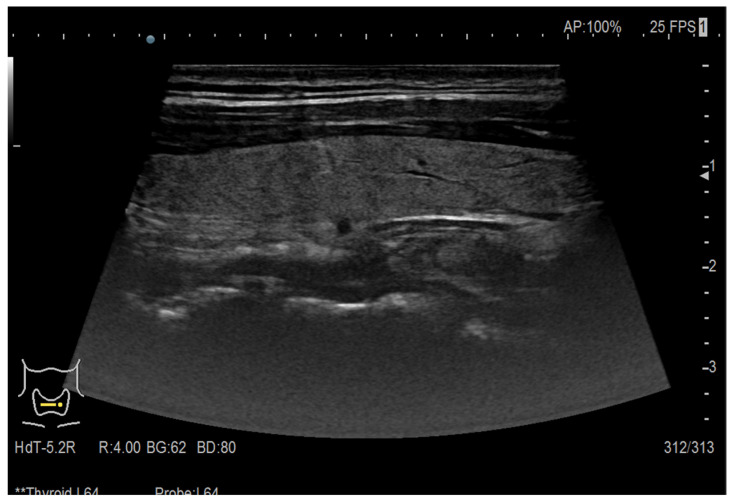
Ultrasound image of a healthy thyroid.
